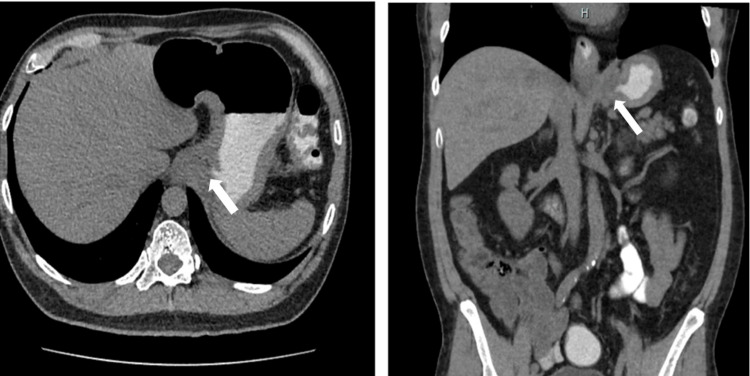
Contrast-enhanced CT of the abdomen axial section (left) and coronal section (right) showing circumferential concentric thickening extending from distal gastroesophageal junction till gastric cardia (white arrow)
